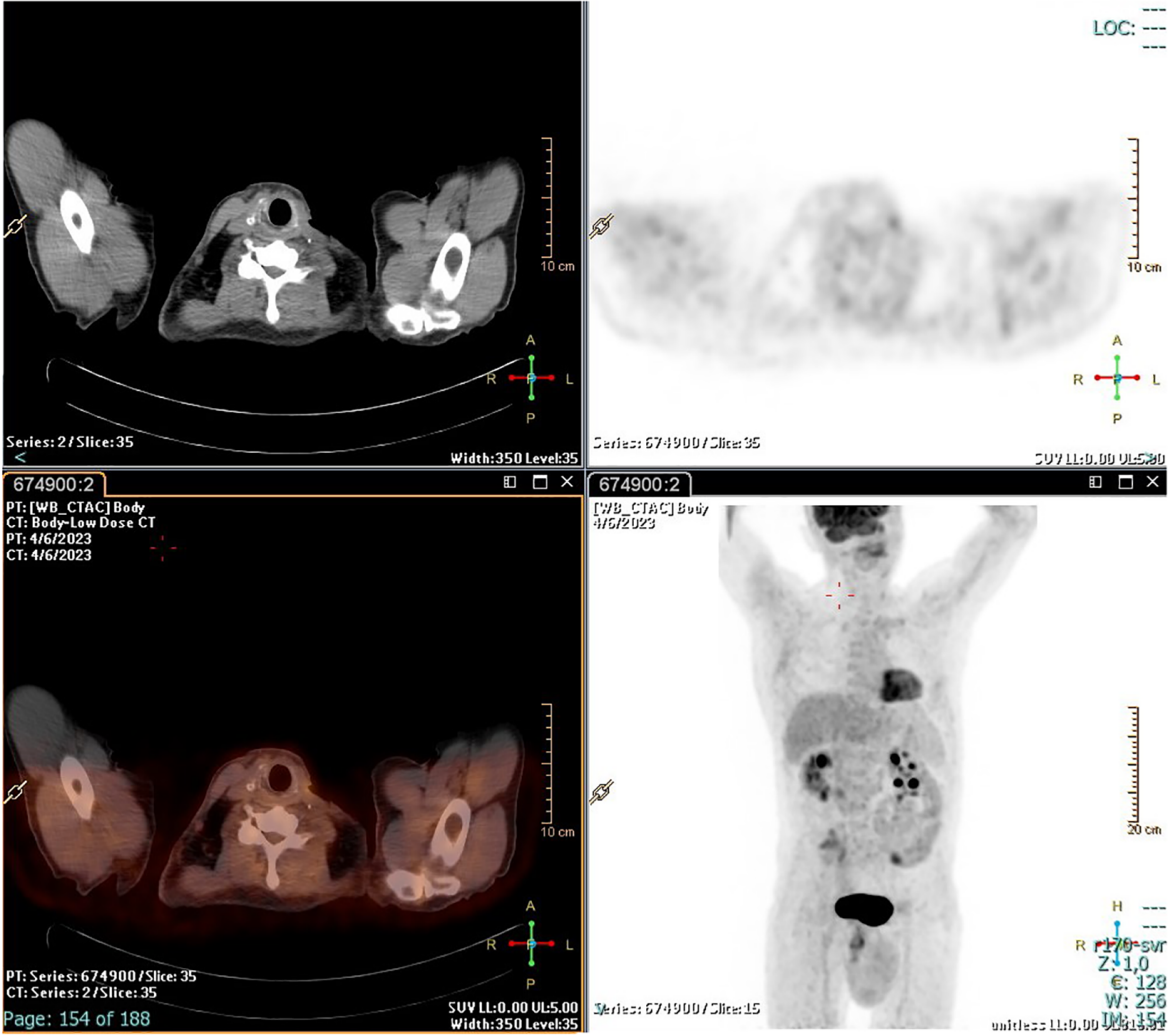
^18^FDG/PET-CT scan after 4 months. No pathological uptake.
